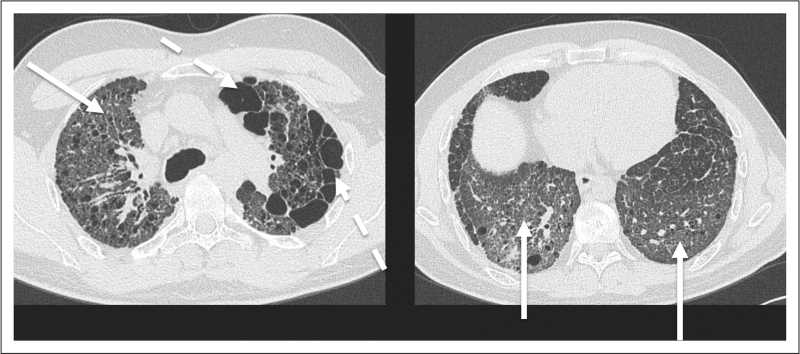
A 41-year-old man with familial pulmonary fibrosis listed for lung transplantation. High-resolution computed tomography shows ground glass opacity (continuous arrows), and paraseptal emphysema (dashed arrows). Both abnormalities predominate in the upper zones. This patient was found to carry a heterozygous *TERC* mutation.
